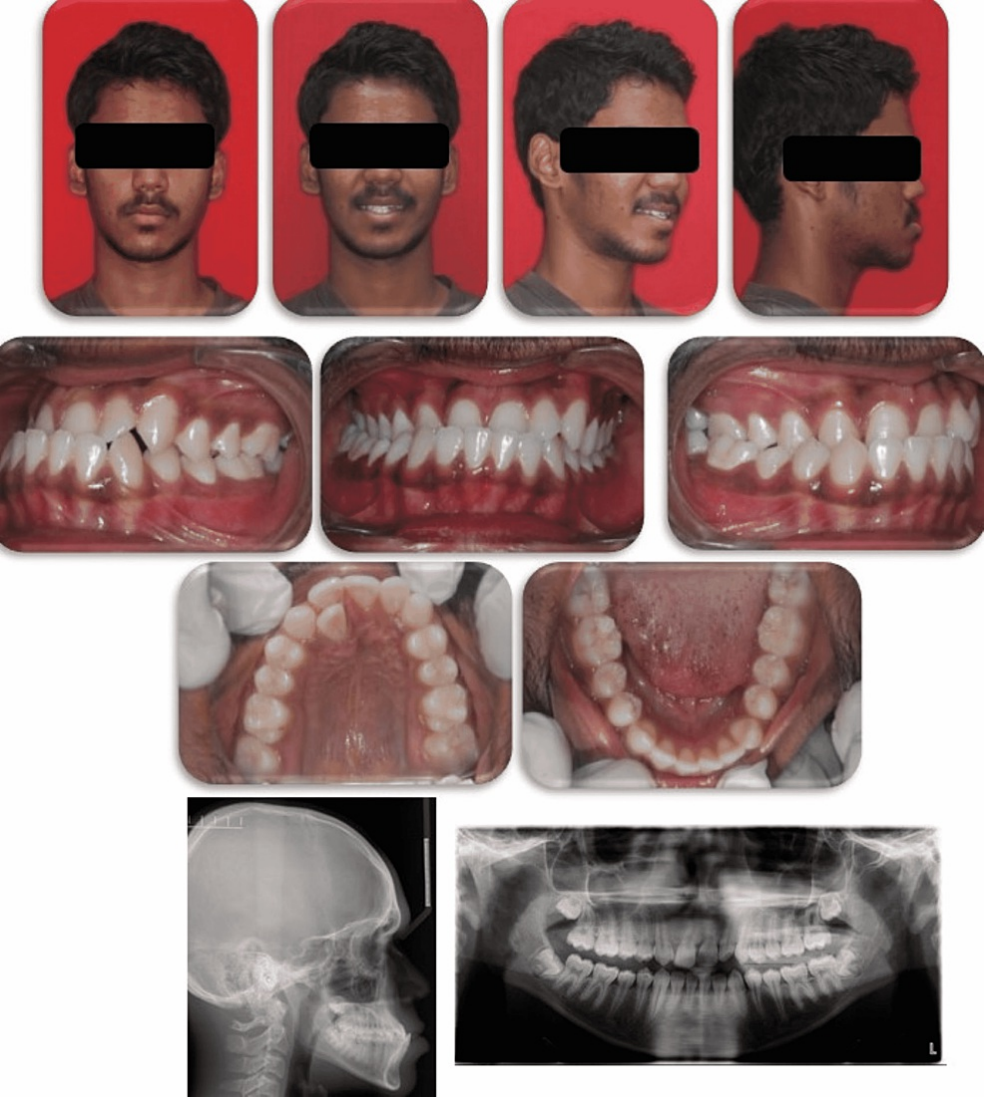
Pre-treatment intraoral and extraoral photographs (lateral cephalogram and orthopantomogram)
